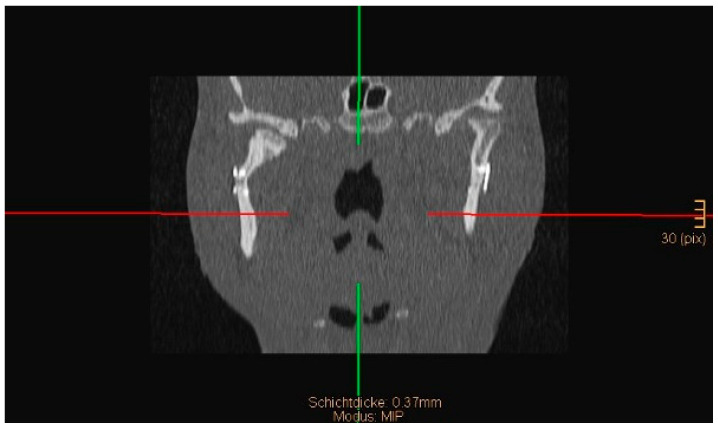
Coronal CT scan showing both condyles after ORIF. A widening of the mandible, caused by a small lingual gap that persisted even after lag screw osteosynthesis at the symphysis, resulted in an angulation of the condylar neck on the right-hand side (same patient as in Figure 3 and Figure 4). The image shown was taken on the third postoperative day after drainage removal and the situation did not worsen over time (last follow-up 1 year postop).
